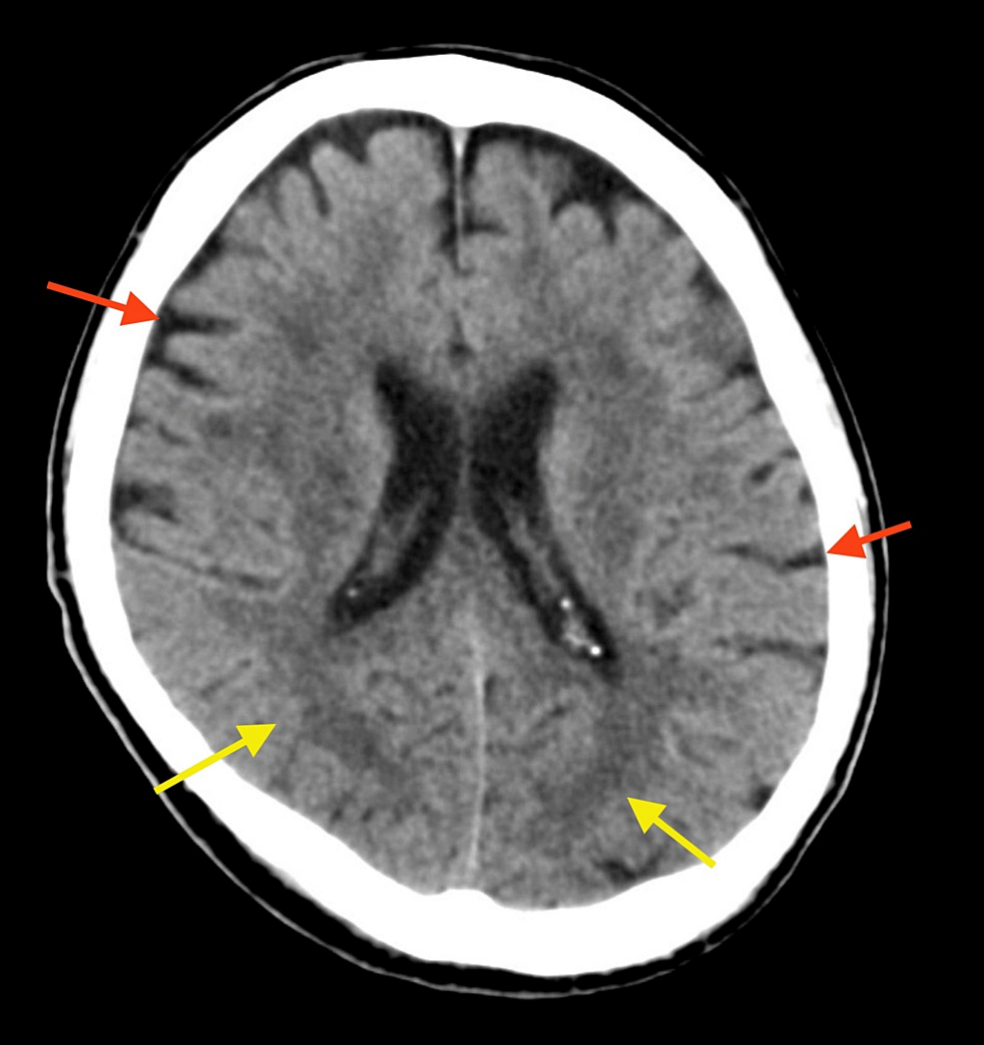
Head CT scan on arrival. The corticomedullary boundary is clear as shown by the yellow arrows, and the cerebral sulcus is not narrowed as shown by the red arrows. CT: computed tomography
